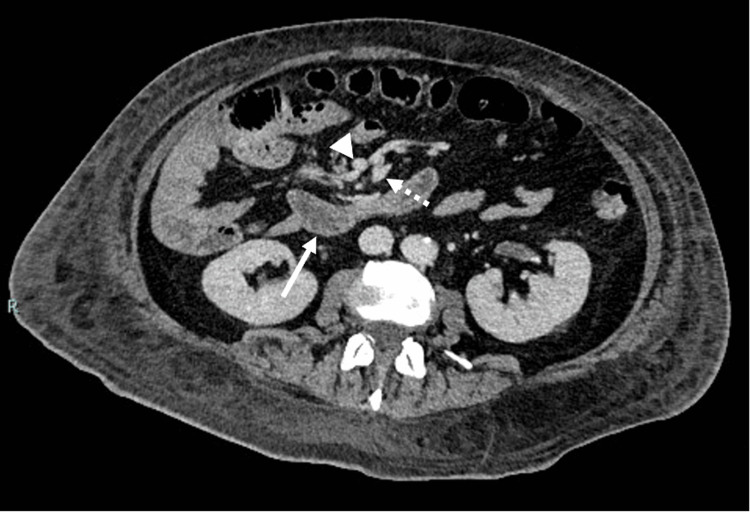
Midgut rotation Contrast-enhanced axial CT image in the venous phase demonstrating clockwise rotation of the second through fourth portions of the duodenum, compatible with reversed midgut rotation (arrow: duodenum; dashed arrow: superior mesenteric artery; arrowhead: superior mesenteric vein).
